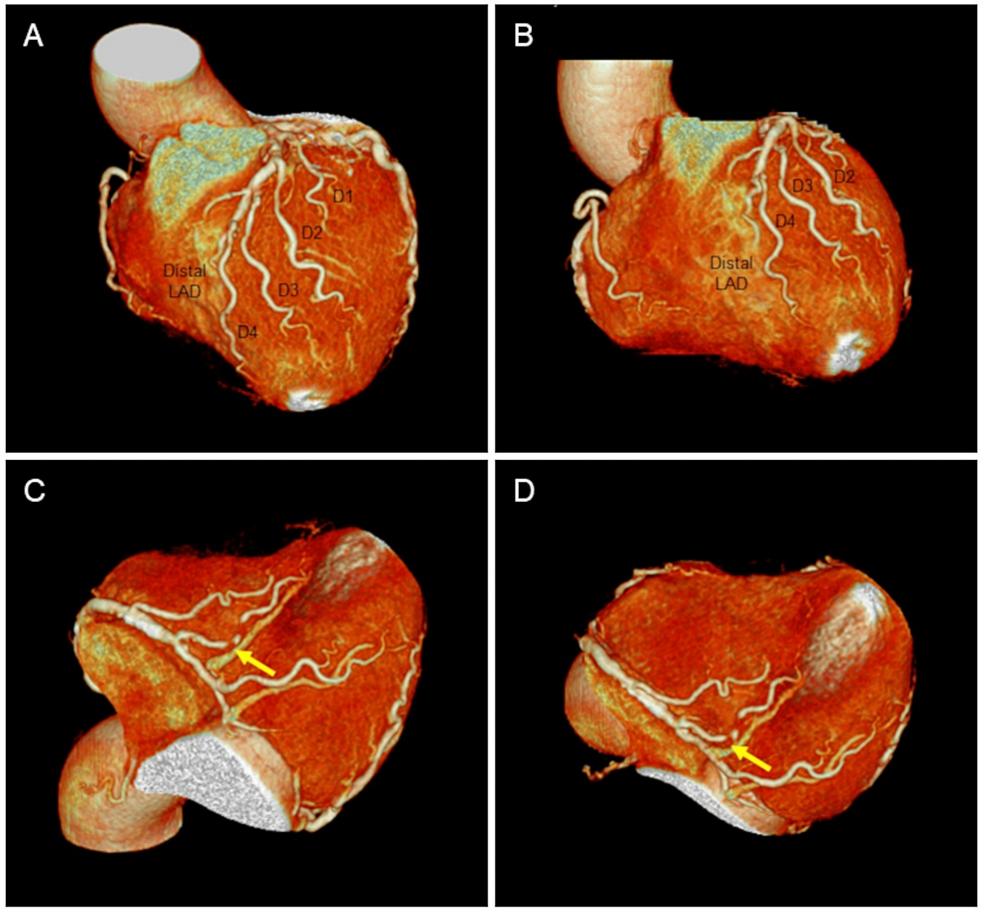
Cardiac CT LAD artery after takeoff of the fourth major diagonal branch (D4) terminated before the LV apex (A and B). As for the right coronary artery, the posterior descending branch was spontaneously recanalized, and its distal end extended toward the LV apex (C and D, arrows). LAD, left anterior descending; LV, left ventricular; CT, computed tomography; RCA, right coronary artery
